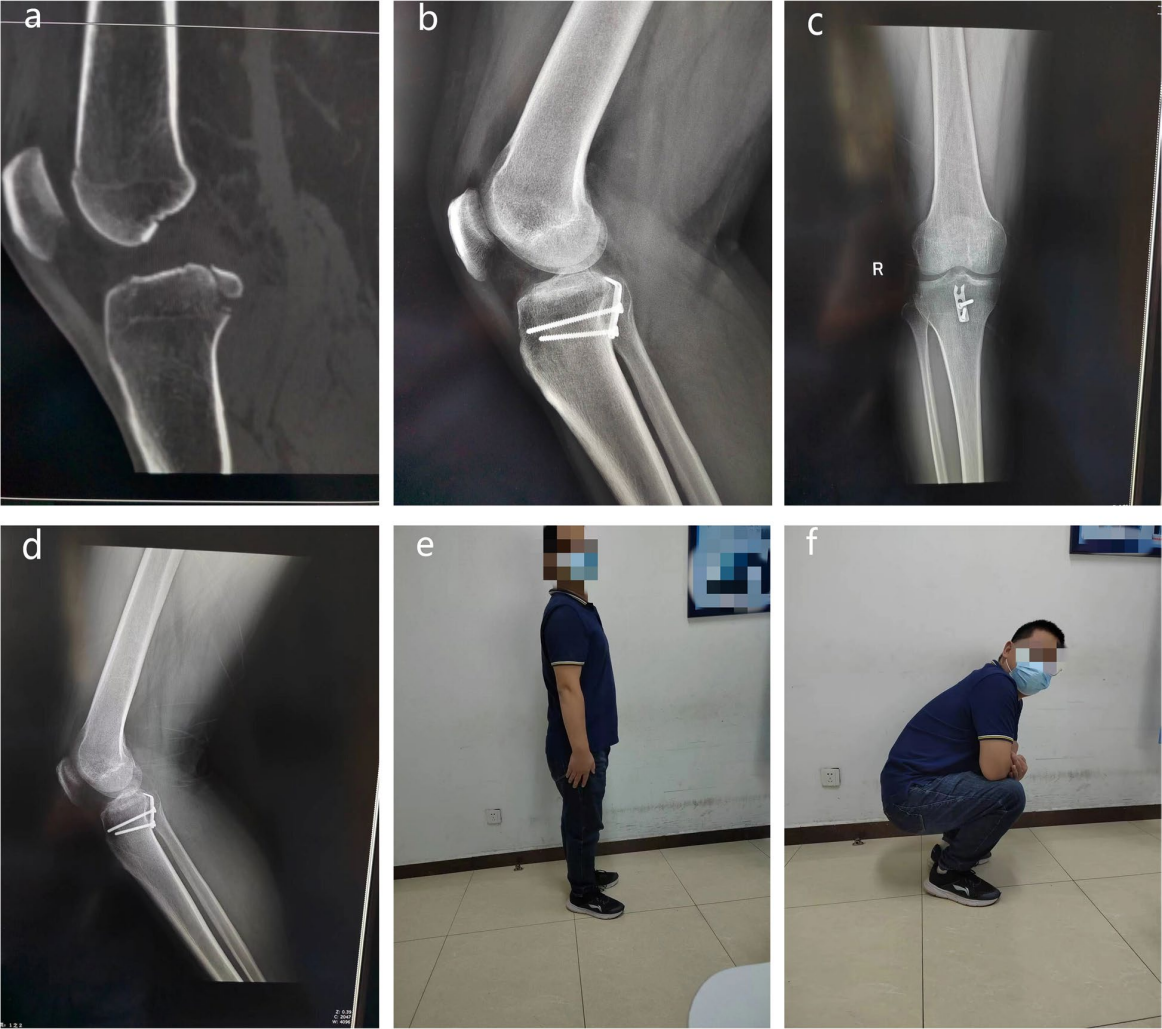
A 32-year-old male presented with an avulsion fracture of the PCL of the right knee. a CT images of the right knee showed PCL avulsion fracture and fragment displacement. b Lateral X-ray film of the knee joint on the first day after the operation. c-d X-ray showing bone healing 3 months after the operation. e-f Photos showing that the patient’s right knee function was good 6 months after the operation
